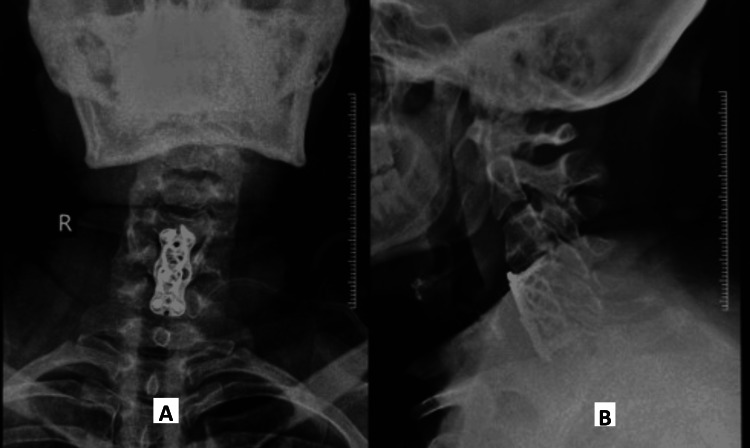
Postoperative day 0 anteroposterior (A) and lateral (B) X-rays showing C5 anterior corpectomy with C4-6 fusion with implant in situ. C4-6: cervical 4-6, C5: cervical 5
